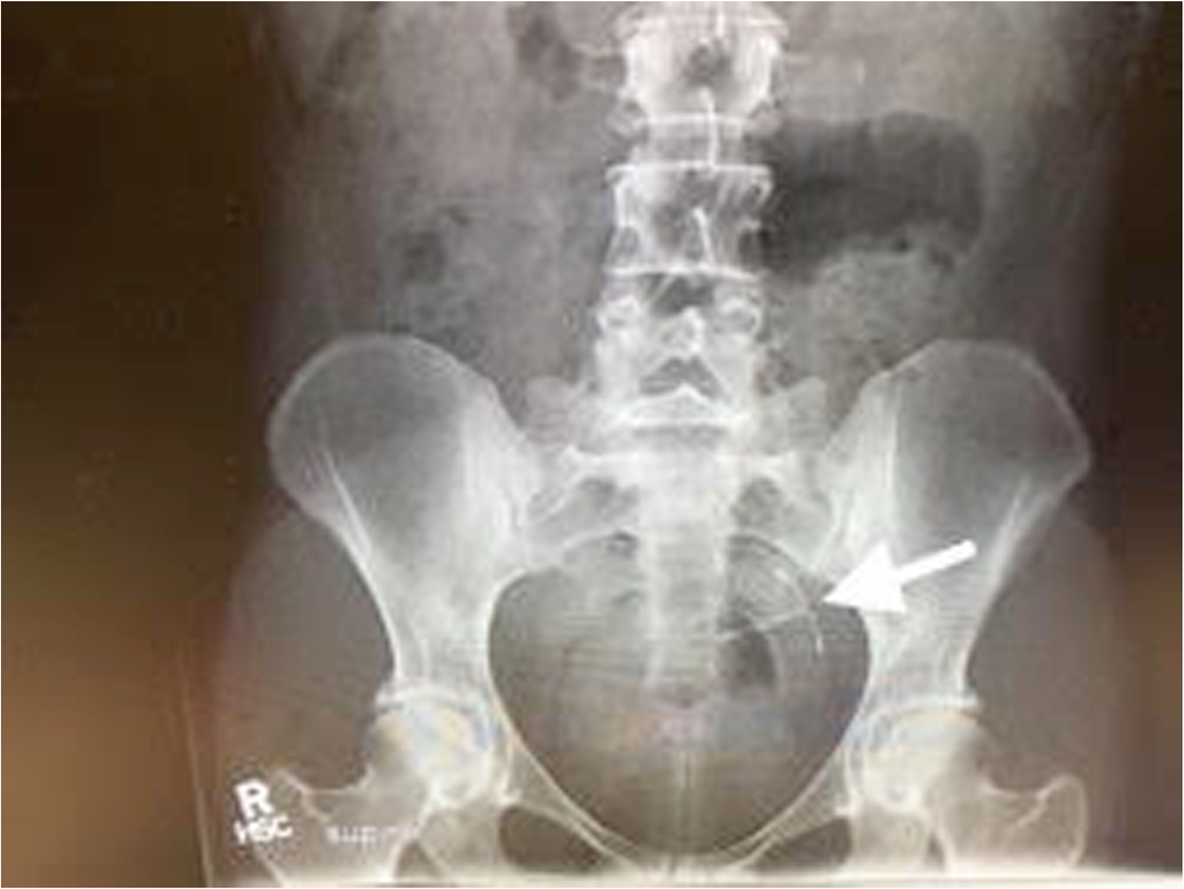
Abdominal radiograph of pelvis with arrow pointing to arms of intrauterine device
